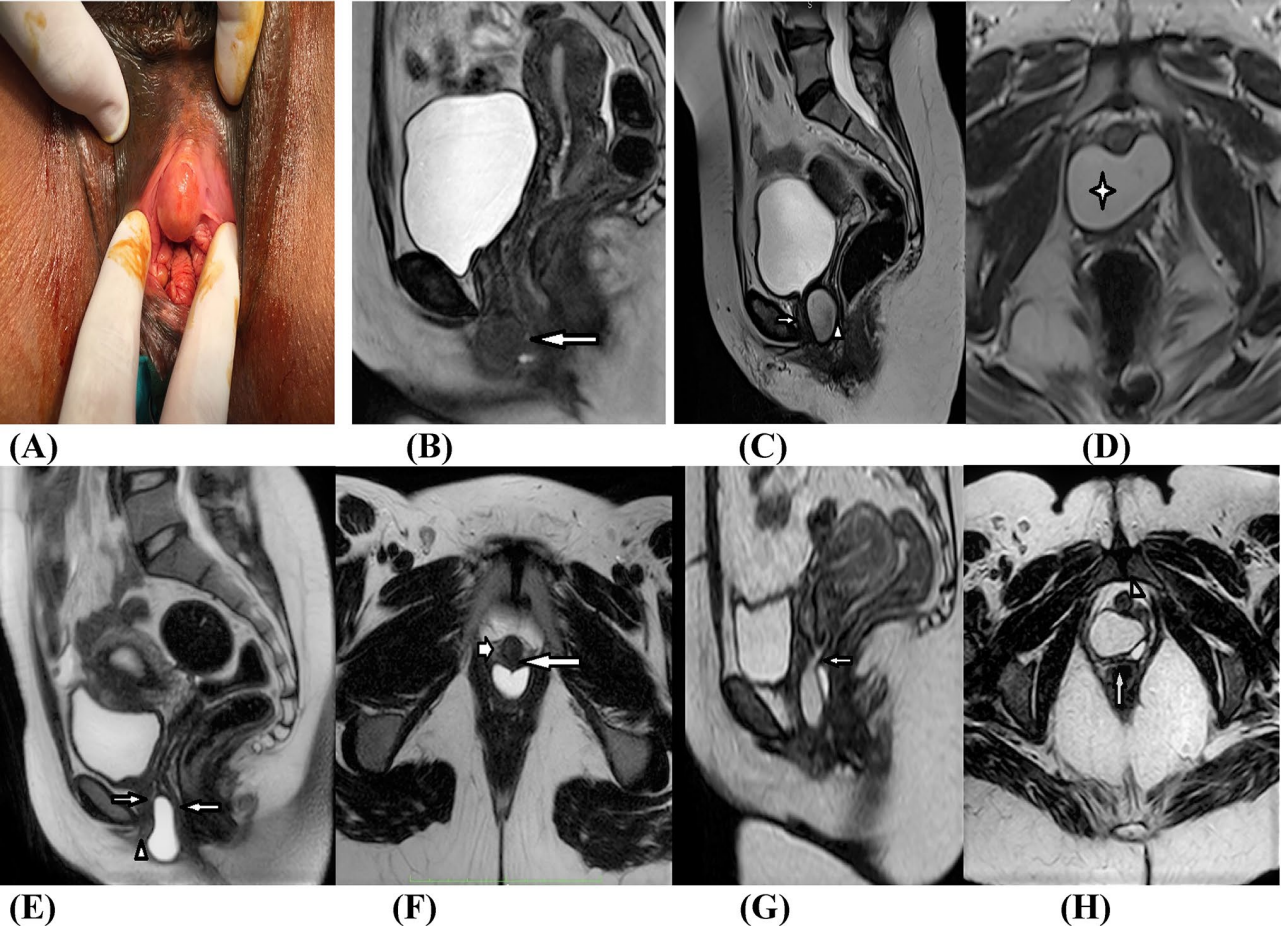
Different cystic periurethral anterior vaginal wall swellings. Skene gland cyst was evident on vaginal examination, with its characteristic juxtameatal location causing eversion of the urethral meatus (A). MRI T2W multiplanar sagittal (B) revealed globular Skene’s gland cyst (arrows in B) centered midline just inferior to the external urethral meatus and the symphysis pubis, and separable from the vagina, slightly effacing it anteriorly with no definite connection to the urethra. It shows signal intensity of concentrated high proteinaceous material notably T2 hypointense and T1 hyperintense. MRI T2W sagittal (C) and T2W axial oblique images (D) revealed a saddlebag cystic structure seen just postero-inferior to the bladder trigone, insinuating between the urethra (arrow in C) anteriorly and vagina (arrowhead in C) posteriorly. The lesion is seen effacing the urethra anteriorly giving the impression of partial encasement from the 3 to the 9-clock axes and pushing on the anterior vaginal wall posteriorly, with no definite connection identified. The cyst contains proteinaceous material with T2 isointense (Asterix in D) and T1 iso- to hyperintense. MRI T2 W sagittal (E) and T2 WI axial oblique (F) images revealed a primary vaginal cystic lesion totally contained within the lower third of the vagina, splaying both anterior and posterior vaginal walls (arrows in E & F), protruding through the introitus effacing the urethra (short arrow in F) and external urethral meatus (arrowhead in E) anteriorly with no connection identified, hence excluding urethral origin. MRI T2 W sagittal (G) and T2 WI axial oblique images (H) revealed a primary vaginal cystic lesion totally contained within the upper two thirds of the vagina and ballooning its lumen, however still with intact surrounding vaginal walls (arrow in H). No connection to the urethra (arrowhead in H) was evident. The cyst harbored clear fluid with no signs of infection. Since a linear vestigial tubular structure was identified connecting the cystic lesion to the vaginal fornix (arrow in), diagnosis of Gartner’s cyst was suggested by MRI
